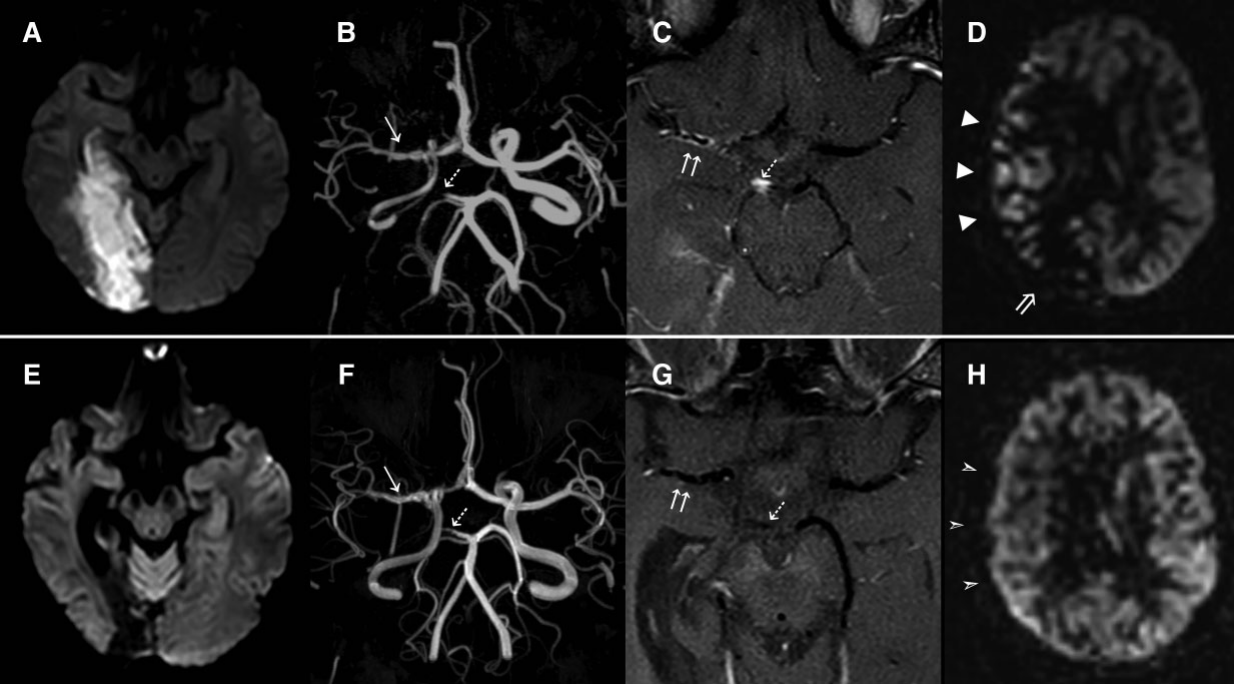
HSV-2 inflammatory intracranial arterial vasculopathy (Patient 7); DWI (A) demonstrates an acute infarct involving the right occipital and medial temporal lobes. ToF MRA (B) shows occlusion of the P1 segment of the right PCA (dashed arrow) and narrowing right MCA with a ‘beaded’ appearance (arrow), as well as diffuse narrowing of the intracranial right ICA. VWI (C) wall thickening and enhancement of the right MCA (double arrow), right P1 segment (dashed arrow) and right intracranial ICA (not shown). ASL (D) shows reduced perfusion in the area of infarction (arrow) and extensive ATAs over the cortical territory of the right MCA (arrowheads), indicating a relative delay in blood arrival. Follow-up imaging after treatment (E-H) shows the right temporo-occipital infarct maturation, with no evidence of new ischaemia on DWI (E). ToF MRA (F) demonstrates partial recanalization of the right PCA (dashed arrow), improvement in the calibre of the right MCA (arrow) and reduced narrowing of the right intracranial ICA. VWI (G) shows near resolution of the wall thickening and enhancement of the right MCA (double white arrows), right P1 segment (dashed arrow) and right intracranial ICA (not shown). ASL (H) shows normalization of perfusion in the corresponding cortical territories of the right MCA and PCA.
